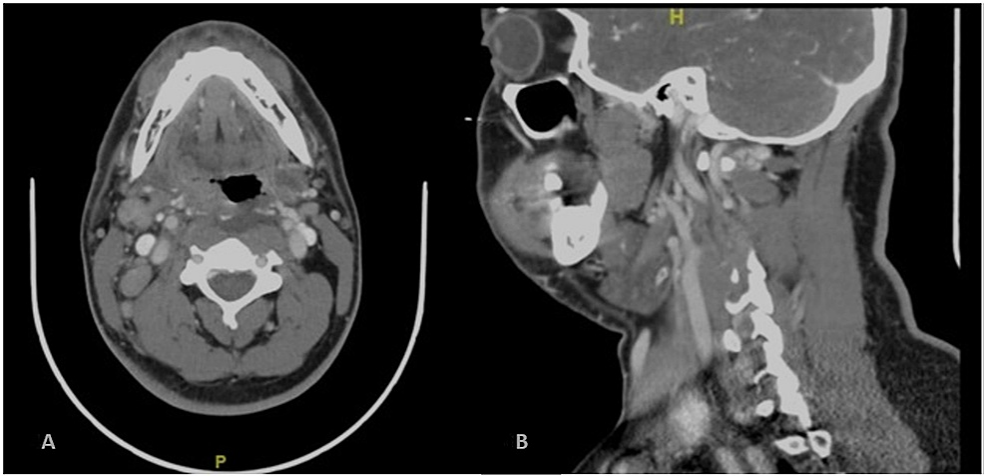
Neck computed tomography (A) Axial section showed a retropharyngeal abscess and enlarged right posterior triangle lymph nodes; (B) coronal image showed a retropharyngeal abscess
